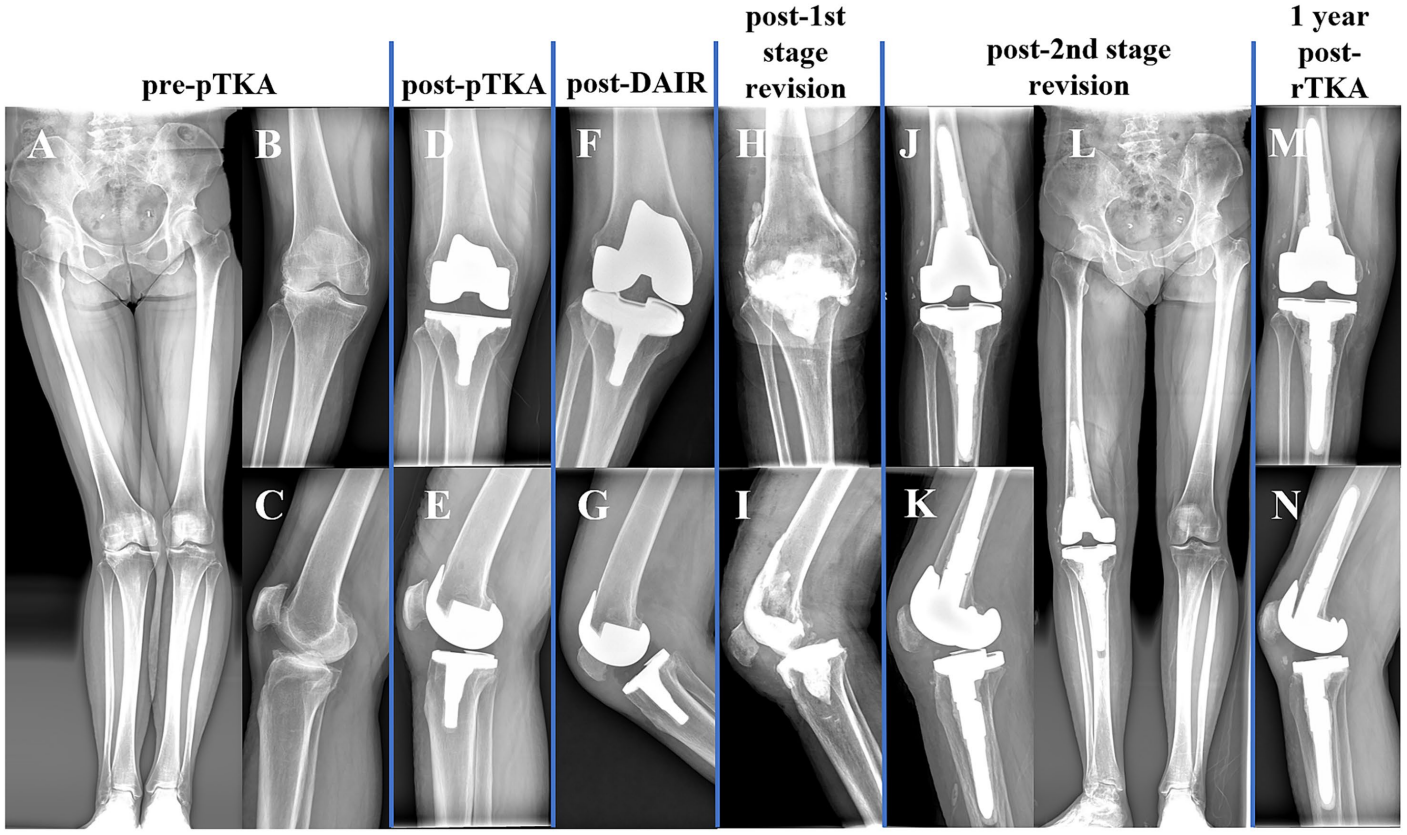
Patient knee joint X-ray images at different stages. (A,B) Before the primary total knee arthroplasty (pTKA); (D,E) Post pTKA; (F,G) Post-debridement, antibiotics, and implant retention (DAIR); (H,I) Post-1st stage revision; (J,L) Post-2nd stage revision; (M,N) One year post-TKA revision.
